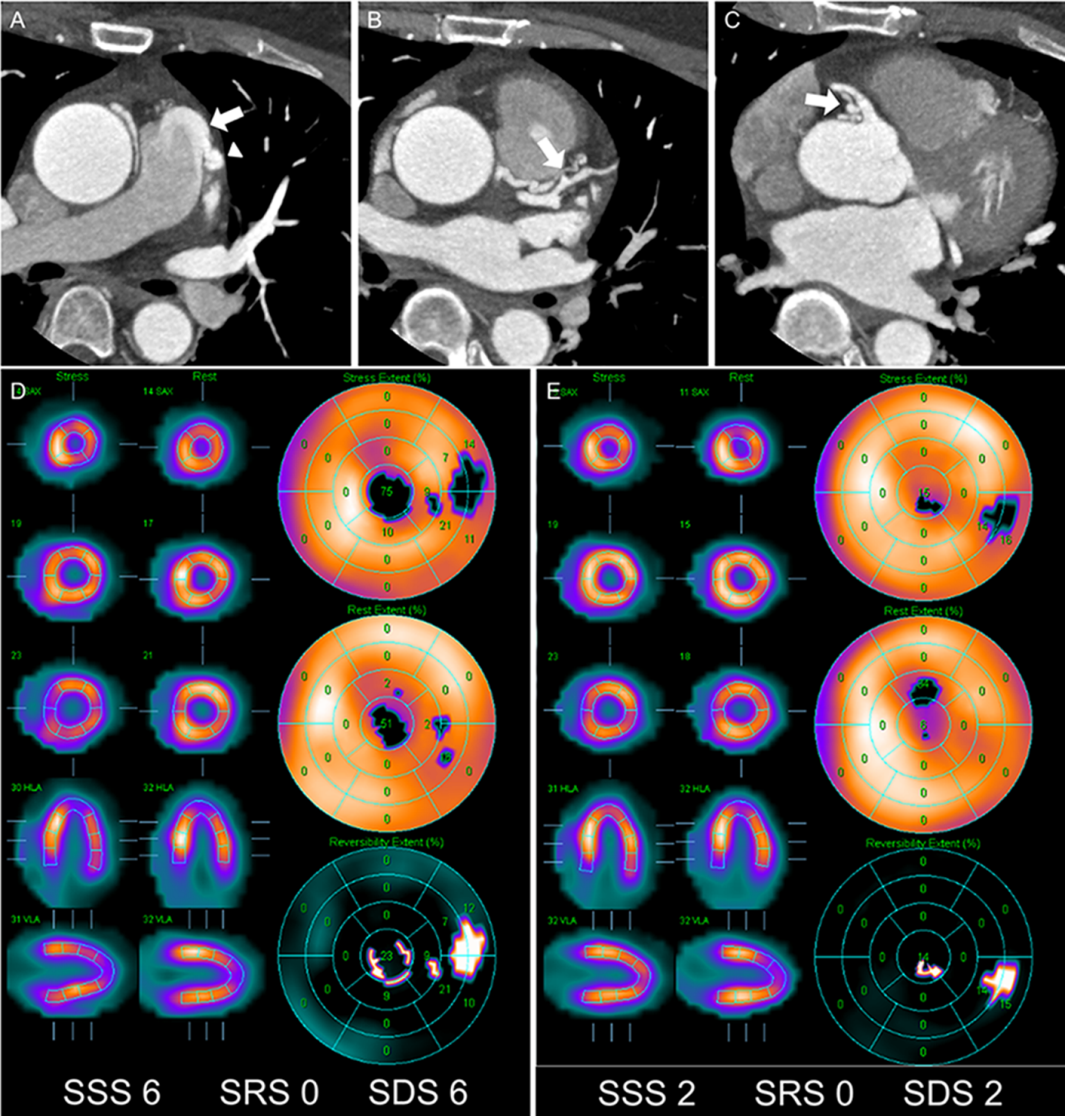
A 52-year-old woman (patient #15) with CPAF who underwent surgical ligation. (A) Axial CCTA images show tortuous and dilated vessels around the main pulmonary artery and a high-density jet flow, which directly inserts into the main pulmonary artery (*arrow*). (B and C) These vessels originate from two different vessels: from the proximal left anterior descending artery (*arrow* in B) and from the proximal right coronary artery (*arrow* in C). (A) This vascular connection passes from the left side of the main pulmonary artery and forms an aneurysmal dilatation (*arrowhead* in A) before it enters the main pulmonary artery. (D) Stress and rest polar maps shows perfusion abnormality (SSS = 6) with moderate reversible ischemia (SDS = 6). (E) After surgical ligation, subsequent SPECT shows decreased extent of the perfusion abnormality (SSS = 2).
